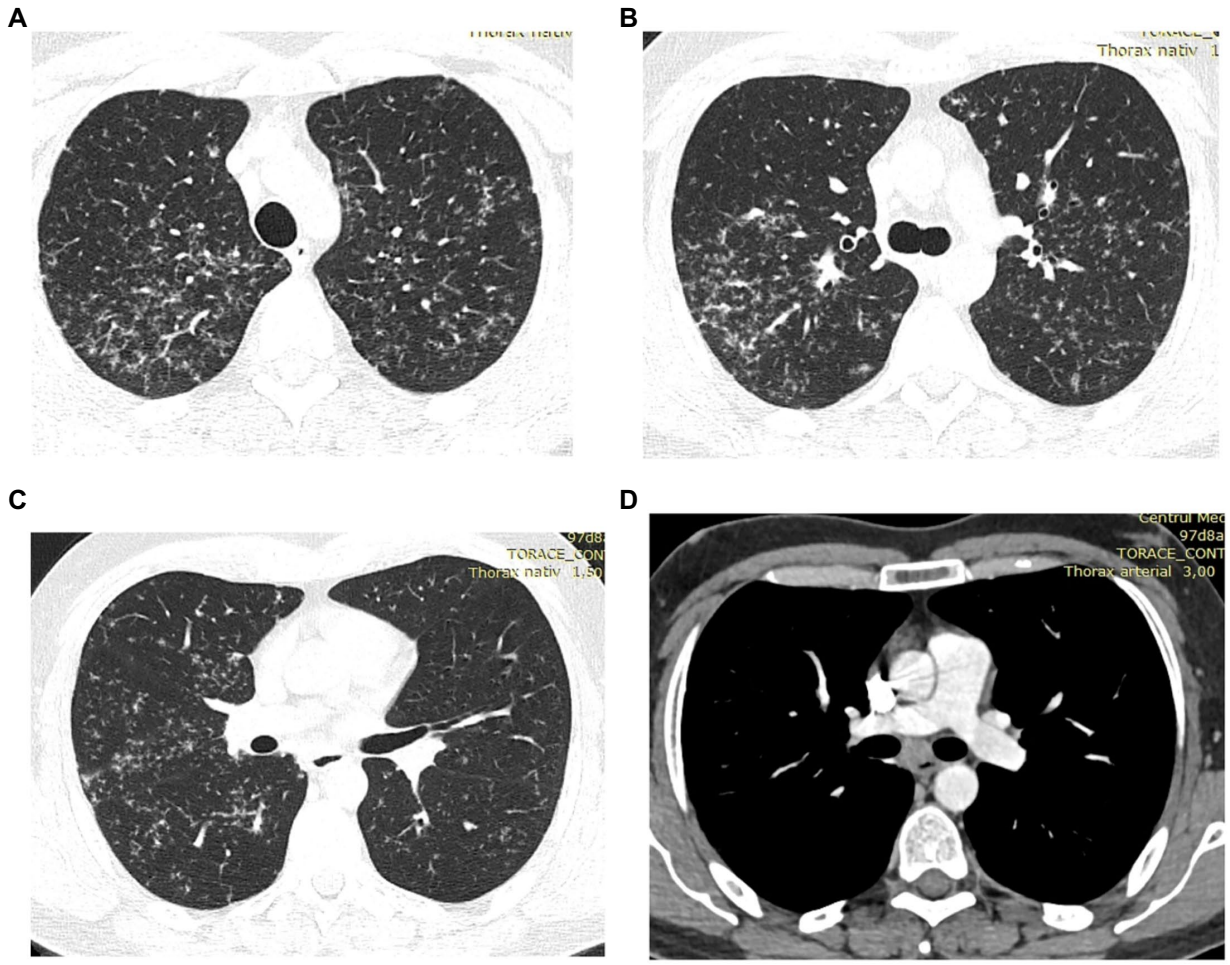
(A–D) HRCT of the lungs: multiple bilateral micronodules predominant in the upper lobes, increased infracarinal lymph nodes.
